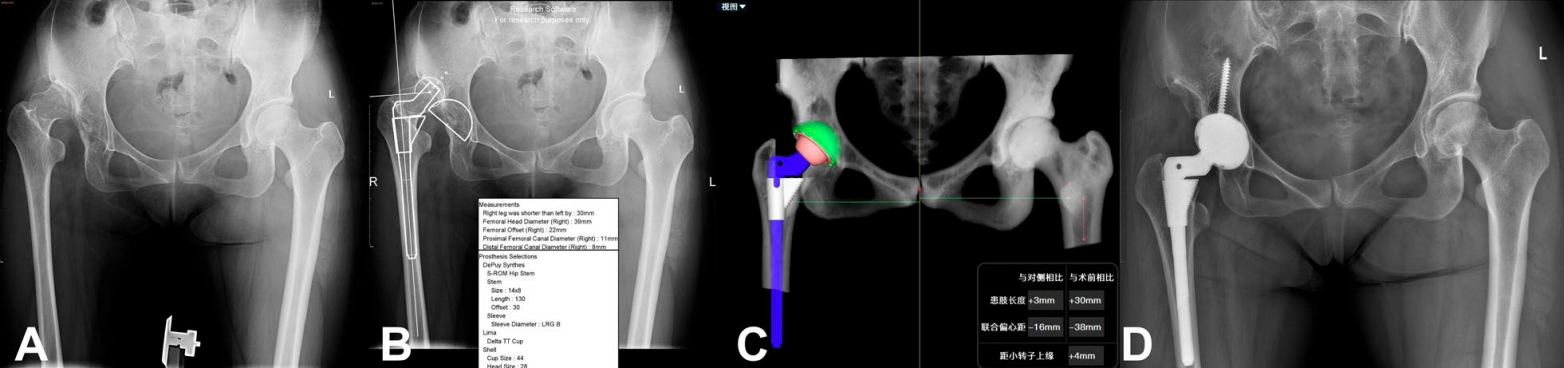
Case 3, woman, 47 years old, Crowe III DDH, right. A Standard preoperative anteroposterior X-ray of the pelvis. B Preoperative planning by the 2D template; planning results: Cup size was 44 mm (Since the OrthoView software does not have a 44 mm Pinnacle cup, a 44 mm Lima cup was planned by the 2D template preoperatively, but the Pinnacle cup was used intraoperatively). C Preoperative planning by AIHIP; planning results: Cup size was 46 mm. D Standard postoperative anteroposterior X-ray of the pelvis: Cup size was 46 mm
